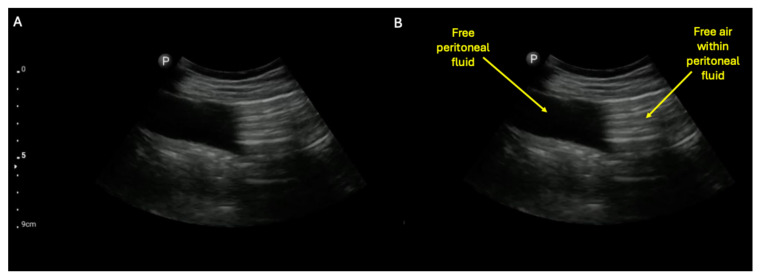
A possible complication of either SBO or ileus is small bowel perforation. The detection of a perforated hollow viscous by ultrasound has been previously described and shown to be diagnostically superior to plain film [14]. A highly suggestive finding is sonographic visualization of pneumoperitoneum within free peritoneal fluid, as shown in the unlabeled (A) and labeled (B) still images (see also Supplementary Video S4 to visualize dynamic movement of the air within the free peritoneal fluid) [14]. The finding of air within free peritoneal fluid is much more suggestive of pneumoperitoneum than just acoustic shadowing within the abdomen, which can be caused by air-filled loops of bowel alone (e.g., see Figure 6 and Supplementary Video S6).
